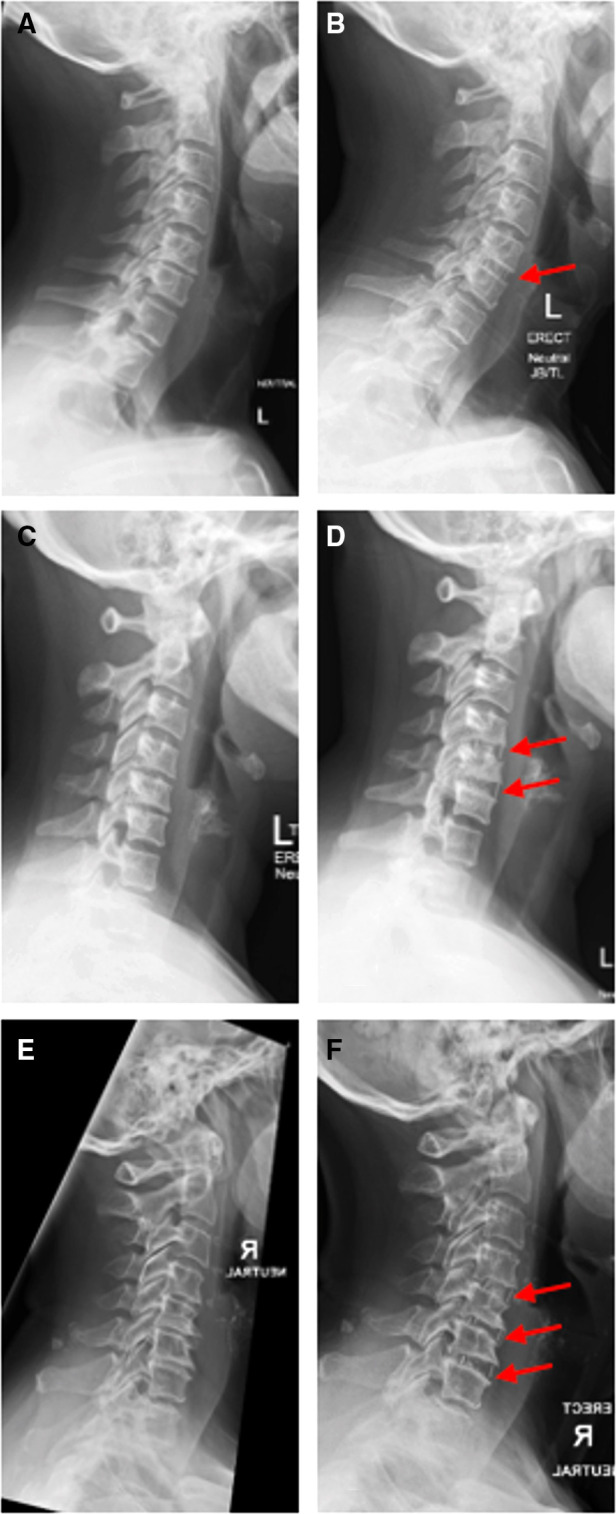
(A,B) Standing lateral x-ray of the true stand-alone cage for cervical degenerative disc disease in one-level (C5/6) preoperatively and at 2-year follow-up. (C,D) Standing lateral x-ray of the true stand-alone cage for cervical degenerative disc disease in two levels (C5/6 and C6/7) preoperatively and at 1-year follow-up. (E,F) Standing lateral x-ray of the true stand-alone cage for cervical degenerative disc disease of three levels (C4/5, C5/6, and C6/7) preoperatively and at 1-year follow-up.
